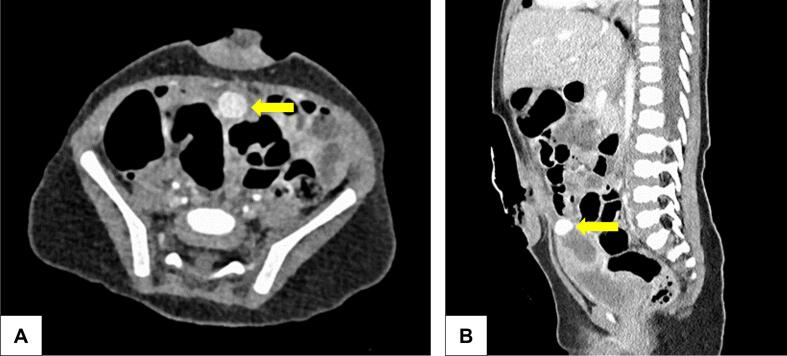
Transverse (A) and sagittal (B) CT abdomen image with contrast medium: A pseudoaneurysm with a maximum diameter of about 1 cm (yellow arrows) is visible behind the umbilical defect cavity. (For interpretation of the references to colour in this figure legend, the reader is referred to the web version of this article.)
